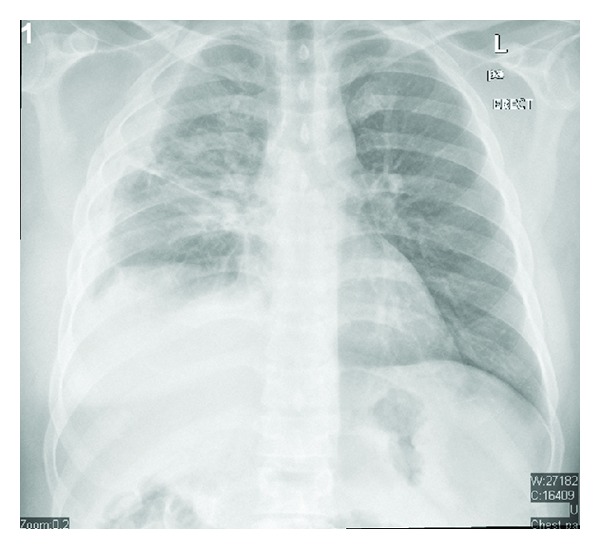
Chest X-ray on discharge.
